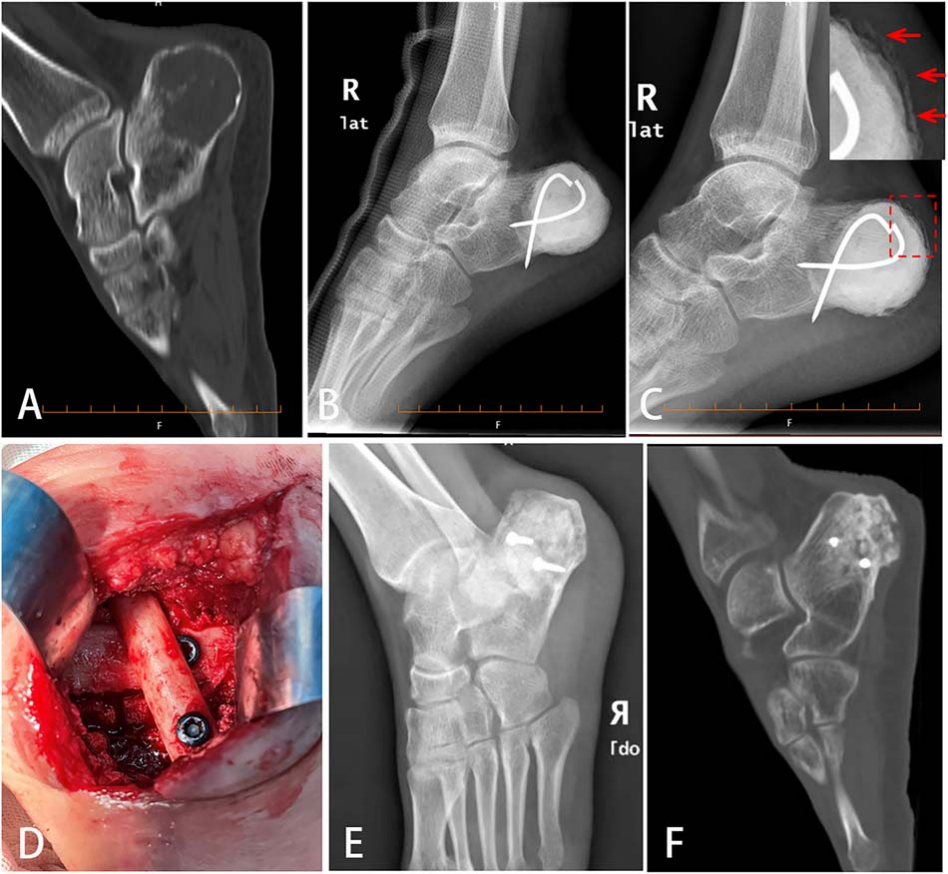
Bone defect reconstruction using Masquelet technique for calcaneal chondroblastoma. (A) X-ray showed osteolytic bone lesion located in posterior of right calcaneus body. The cortex was thinned because of tumor aggression. (B) After tumor was removed totally, bone cement (PMMA) was filled into the cavity and fixed with two cross Kirschner wires. (C) Six weeks later, the induced membrane on the calcaneal surface could be seen obviously (indicated by arrow). (D) Autologous fibular segment shoring and bone graft were filled into the cavity to reconstruct the defect. (E，F) Eleven months later, DR (E) and CT (F) scan showed good bone healing.
